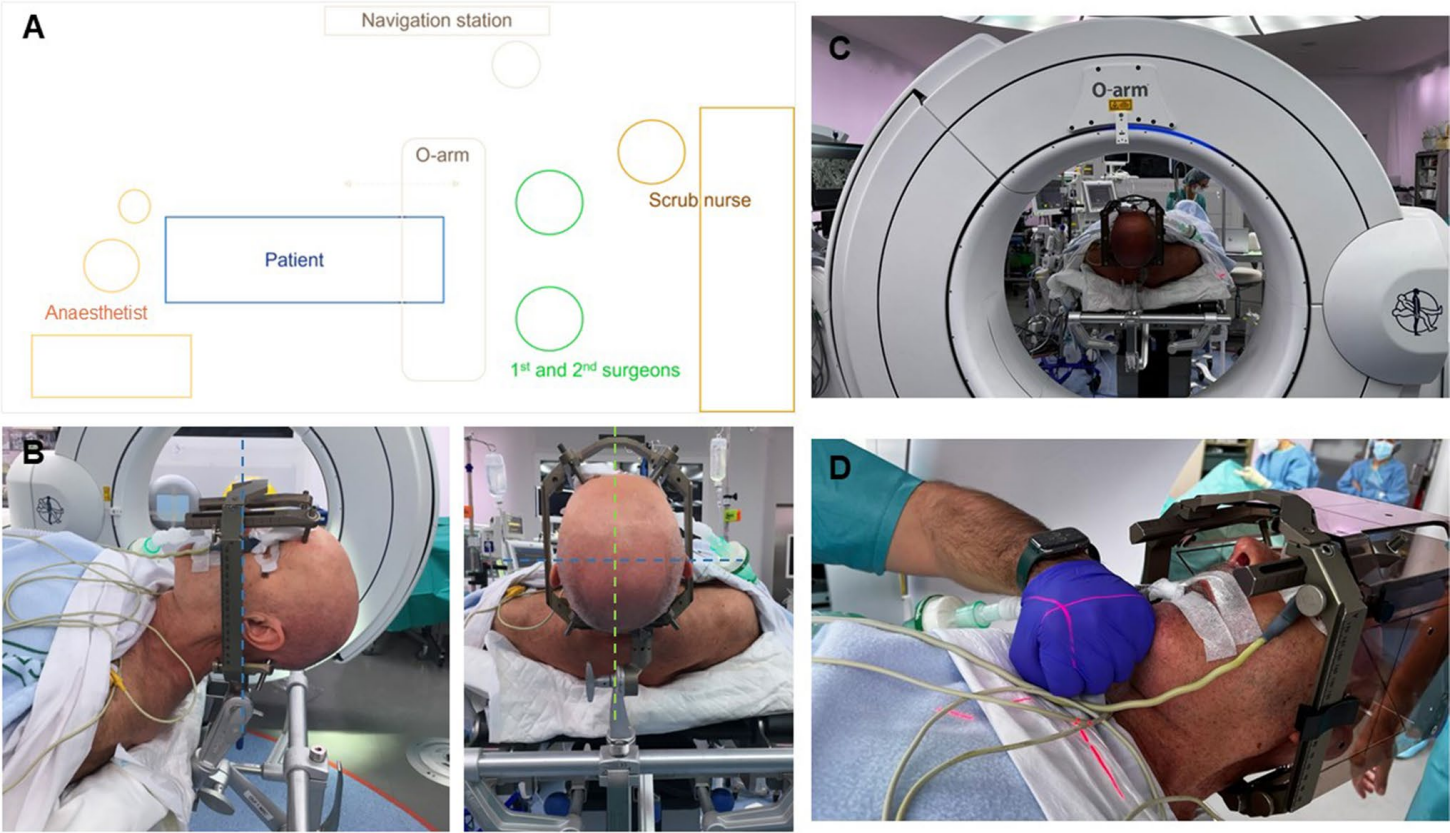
Room setting and patient positioning. A Standard operating room set up. B Stereotactic frame placement, centred on the midline (green dashed line) and parallel to the intermeatal line (blue dashed line). C The head-holder fixation arm should be as packed as possible, to facilitate O-arm movements. D The O-arm position is adjusted, so that the laser lights coincide with the midline, the external acoustic meatus and about one fist below the chin to include the whole skull base. The parameter for CT acquisition are set (supine position, 3D, stereotactic, 120 kV, 20 mA, 40 cm field, 150mAS)*,* and the examination and parking positions are established. After the first CT is acquired, the O-arm is moved to the parking position, and the surgical site is draped and prepared
